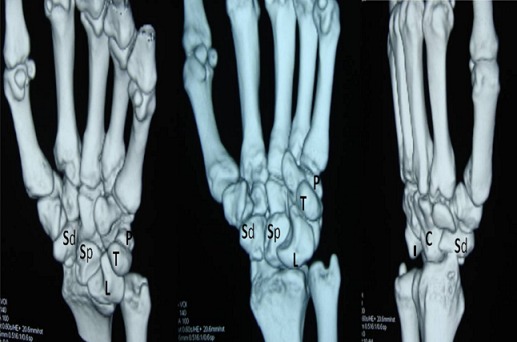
(a),(b),(c): Computed tomography 3D images showing dislocated proximal row scaphoid (Sp), lunate(L), triquetrum(T) and pisiform(P) pushing the capitulam(C)
